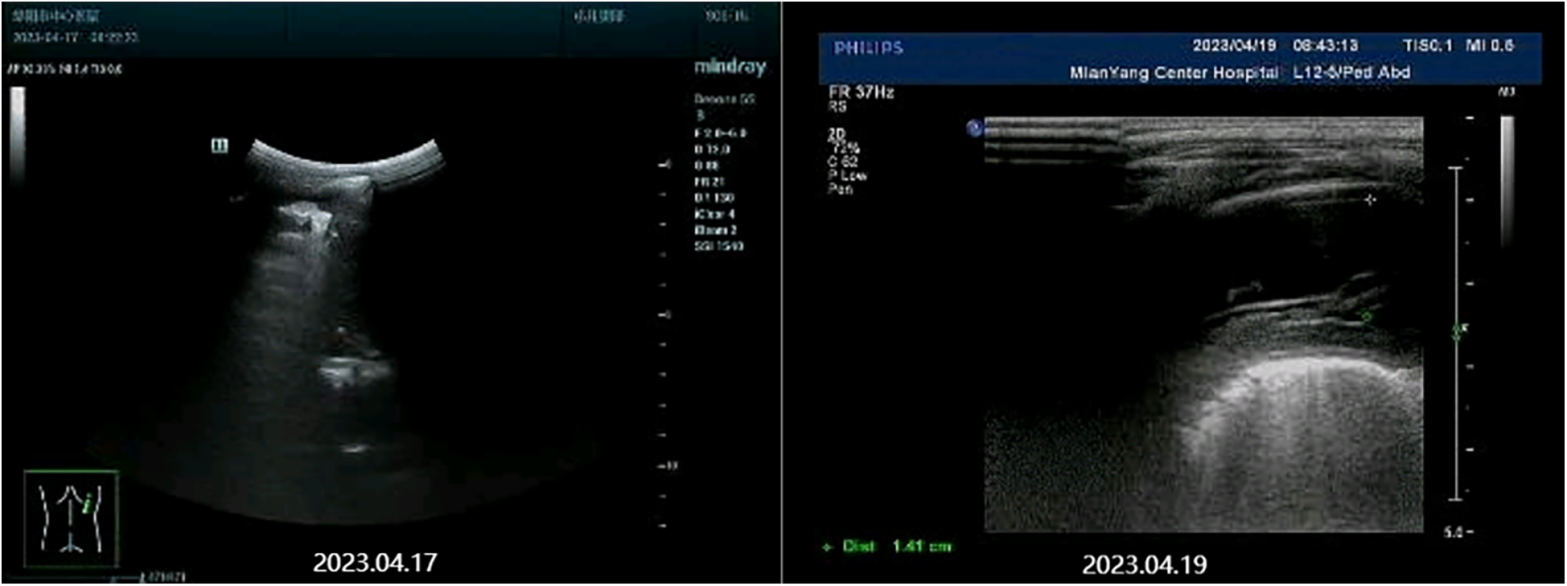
Chest color ultrasound images after treatment.
